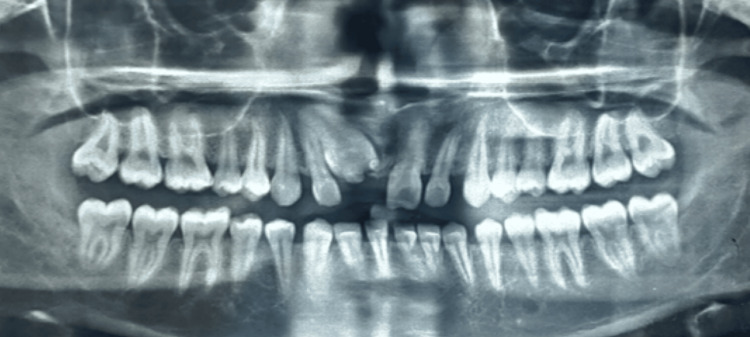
OPG Showing supernumerary teeth along with impacted central incisor in the upper right quadrant OPG: Orthopantomogram
